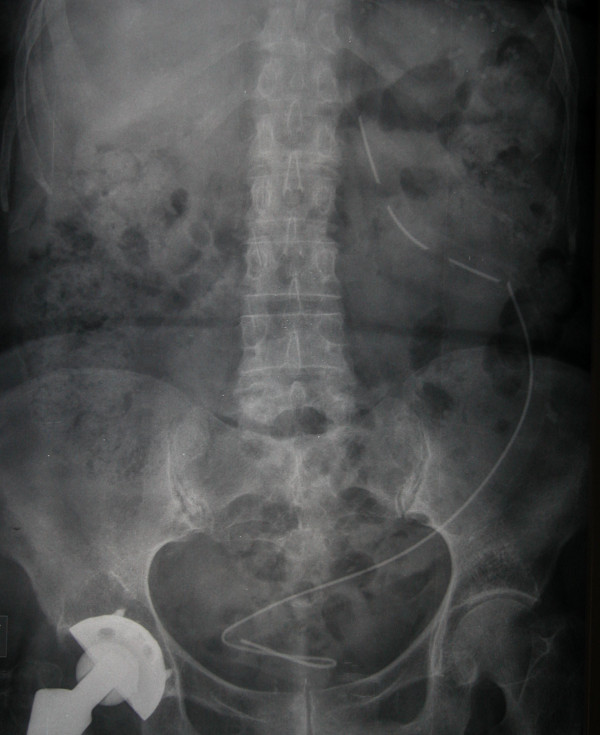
Abdominal X-ray showing a radiopaque stent in the left collecting system: the upper extremity is apparently uncoiled and there are two short interruptions at the proximal third, while the lower extremity forms a wide coil inside the bladder.
